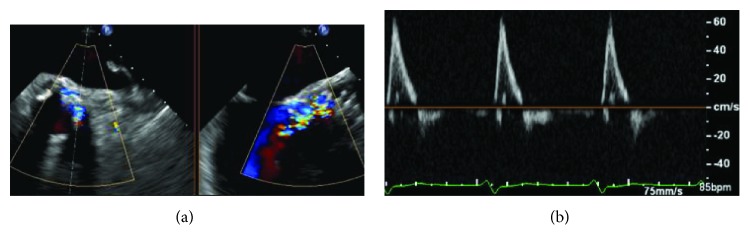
(a) Transoesophageal echocardiography revealing the sealing of the paravalvular leak and the presence of a mild residual aortic regurgitation after the deployment of a 29 mm EDWARDS Sapien 3. (b) Pulsed wave Doppler in the descending aorta showing an antegrade flow without diastolic flow reversal.
